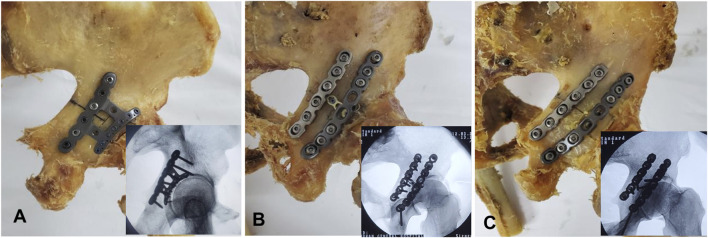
Photographs and radiographic imaging showing the three configurations used for APCWF fixation. (A) an anatomically integrated plate; (B) 6-hole and 8-hole 3.5 mm conventional reconstruction plates combined with a T-plate; (C) 6-hole and 8-hole 3.5 mm conventional reconstruction plates combined with two lag screws.
